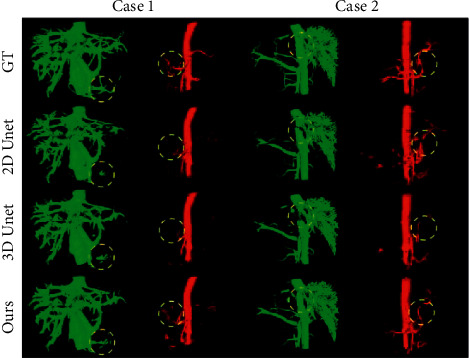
Results of segmentation of arterial and venous vessels on venous phase CT. Representative blood vessels are shown in the yellow dotted circle, and the position shown in the third column is the junction of the inferior vena cava and the liver.
